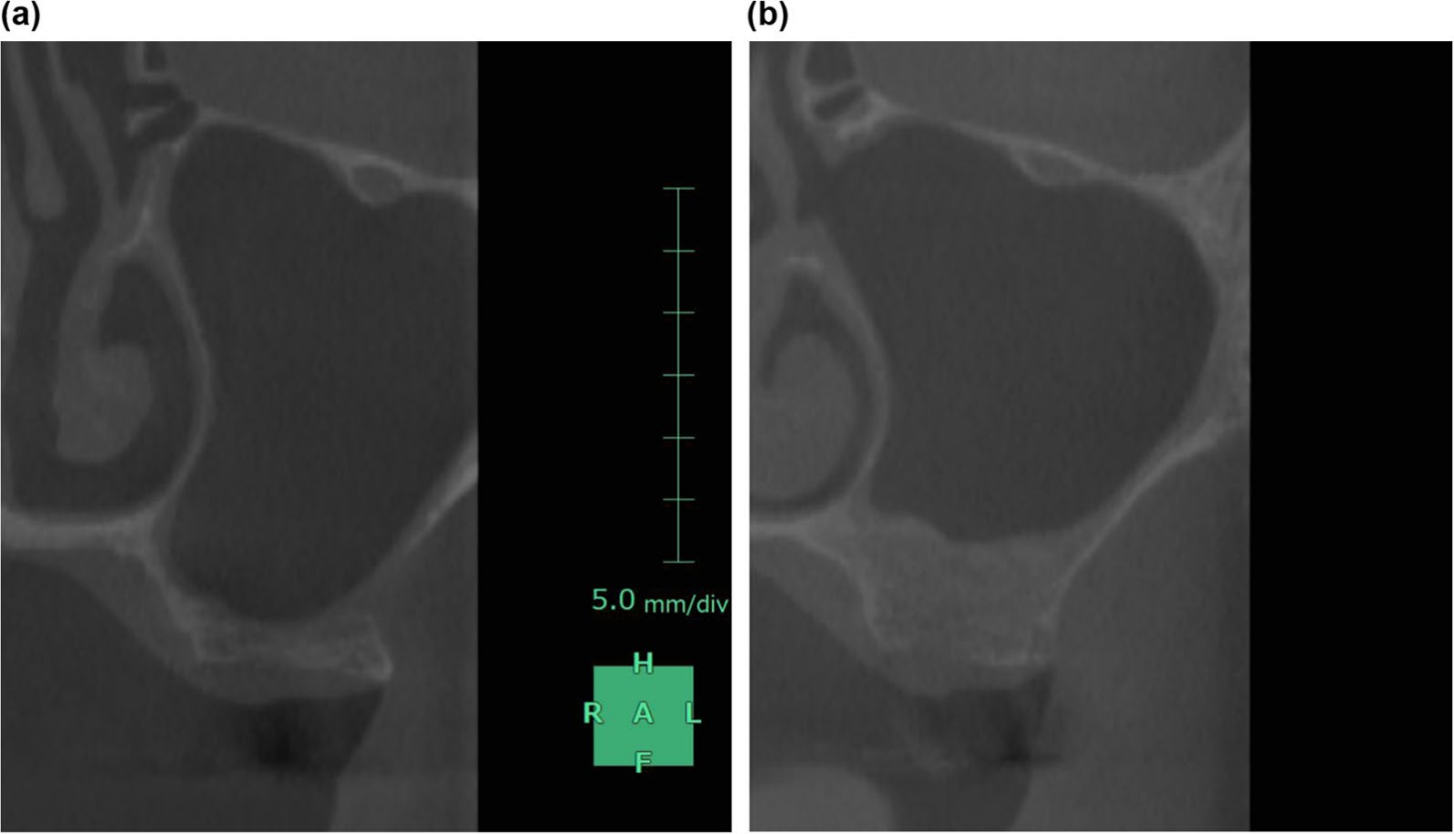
a Preoperative cone-beam computed tomography (CBCT) data. b Postoperative CBCT data
